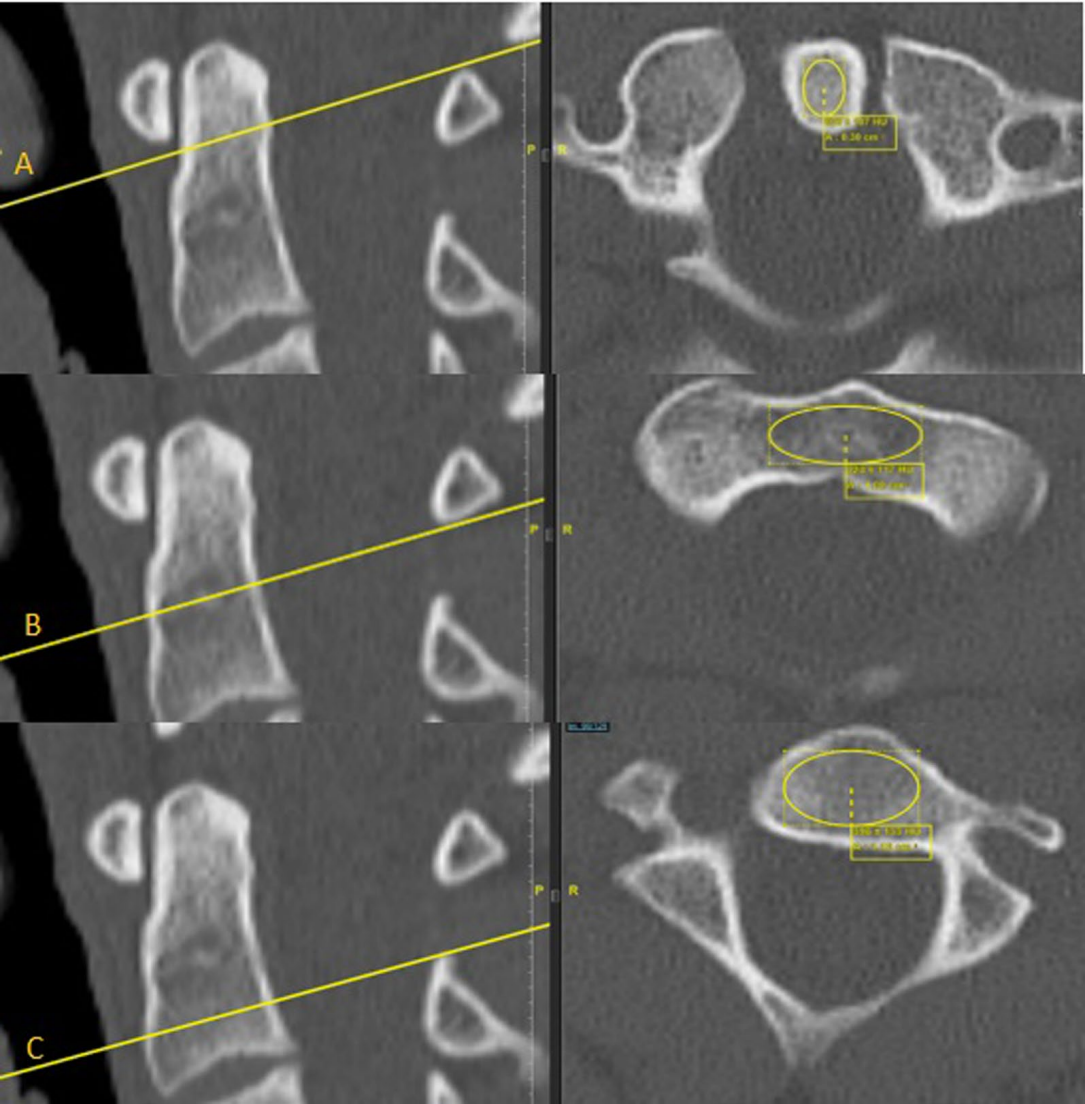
Determination of HU of the 2nd vertebra in 3 regions: Dens axis (A), transition area (B) and vertebral body (C) using computed tomography
